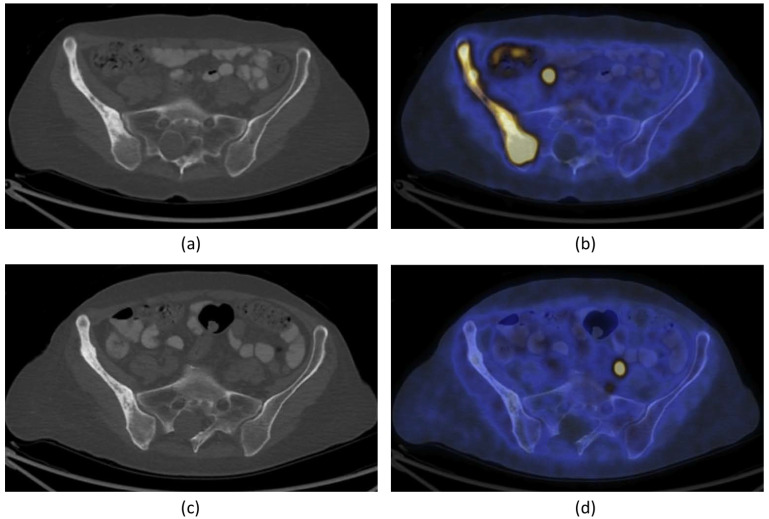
A 66-year-old woman with bone metastasis in the right ilium. Trans-axial ^18^F-FDG PET/CT slide at the level of the pelvis (a): CT; (b): fused PET/CT) demonstrates pathological increase in ^18^F-FDG uptake in a larger mixed lytic-sclerotic lesion before treatment. After MR-HIFU treatment, no ^18^F-FDG uptake was observed in the right ilium (d). There is, however, no change in the bony structure on CT (c). The findings are consistent with healed metastasis in the right ilium with no evidence of active residual malignancy in the site. FDG = fluorodeoxyglucose; PET = positron emission tomography; CT = computed tomography). Figures adapted and reprinted from Journal of Pain and Symptom Management, Volume 56 Issue 1, Eisenberg E, Shay L, Keidar Z, Amit A, and Militiau D, Magnetic Resonance–Guided Focused Ultrasound Surgery for Bone Metastasis: From Pain Palliation to Biological Ablation?, 158–162, 2018, with permission from Elsevier (Amsterdam, The Netherlands) [32].
